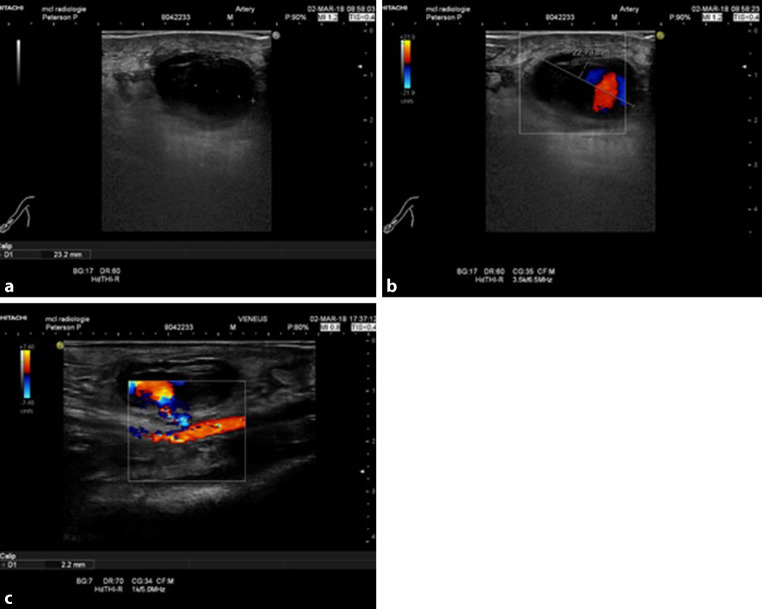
a Doppler ultrasonography showing pseudoaneurysm of right radial artery. b Echography with colour. c Flow from radial artery into pseudoaneurysm
